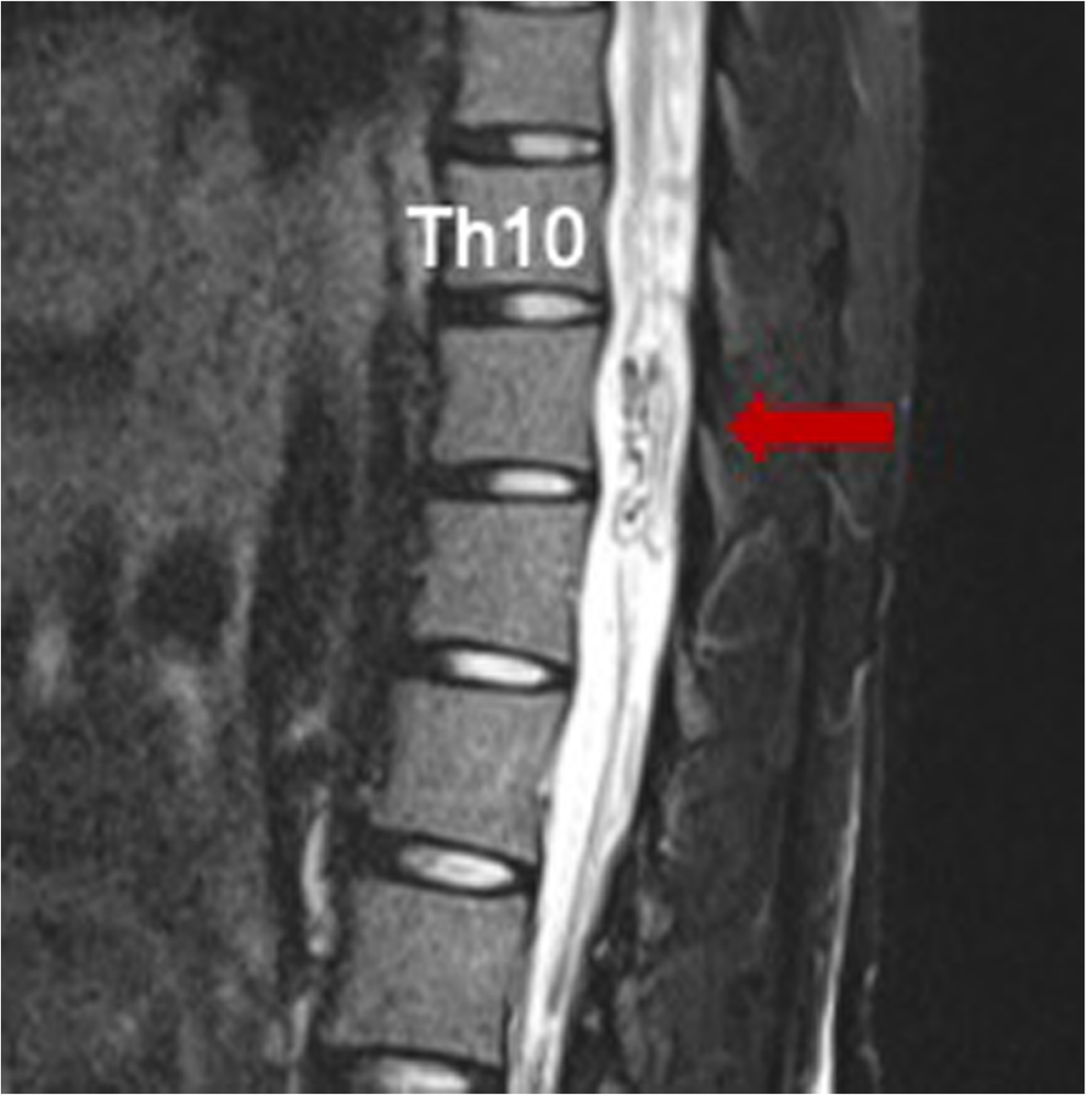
Magnetic resonance imaging (T2-weighted image) in the thoracic level at 32 weeks of gestation. Flow void (the arrow) suggests a dilated draining vein. Th10, tenth thoracic vertebra
